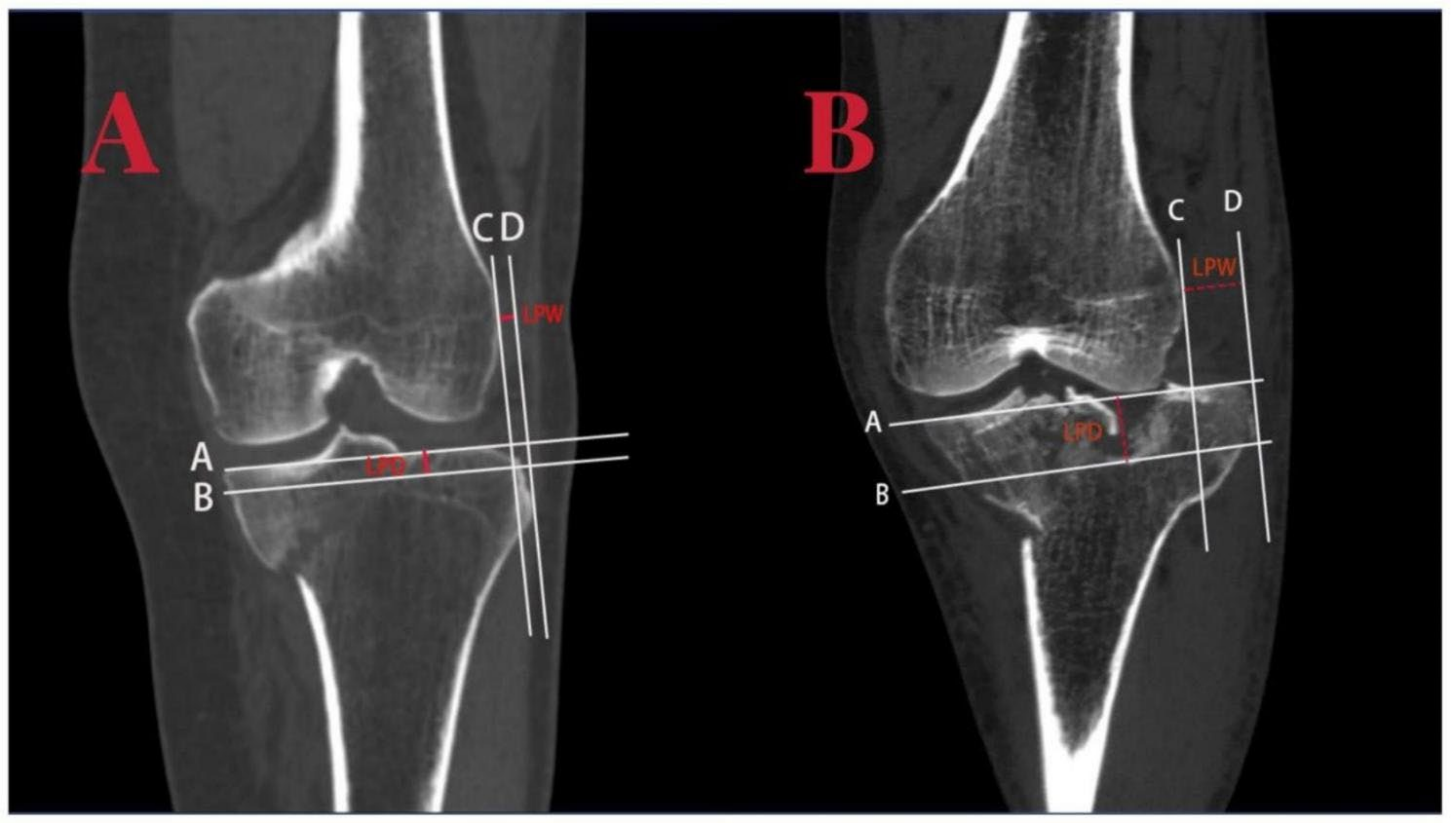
Measurement of lateral plateau depression (LPD) and lateral plateau widening (LPW) in the CT Coronal Scan. Example A: A 52-year-old male patient with a Schatzker IV-C Tibial Plateau Fracture and no lateral meniscus injury; the LPD measures 7.84 mm and the LPW measures 4.65 mm. Example B: A 50-year-old female patient with a Schatzker IV-C Tibial Plateau Fracture and lateral meniscus injury; the LPD measures 20.50 mm and the LPW measures 12.68 mm
